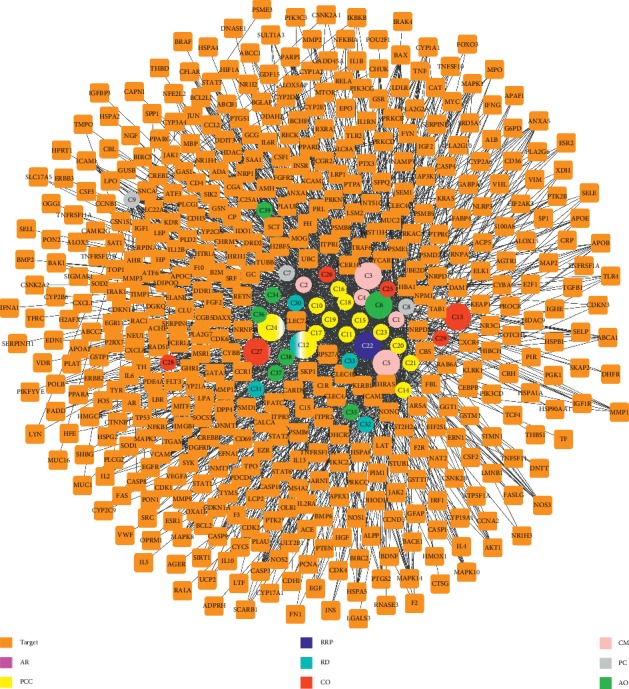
CT network. The multicolored circles represent compounds of different herbs (purple for AR, yellow for PCC, dark blue for RRP, cyan for CO, red for RD, pink for CM, grey for PC, and green for AO) and orange squares represent the targets of each compound. One target can have multiple compounds and vice versa. AR, Anemarrhenae Rhizoma; PCC, Phellodendri Chinensis Cortex; RRP, Rehmanniae Radix Praeparata; RD, Rhizoma Dioscoreae; CO, *Cornus officinalis*; PC, poria cocos; AO, *Alisma orientale*; CM, Cortex Moutan.
